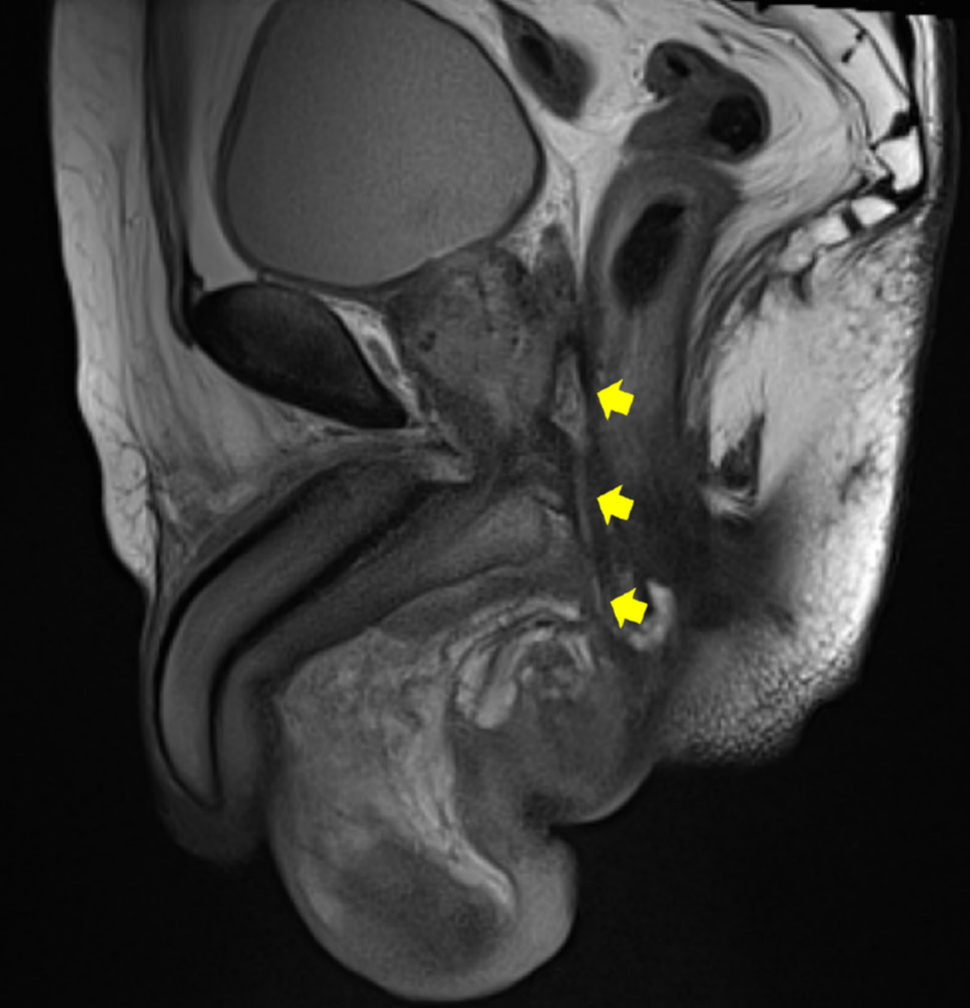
MRI showed tunnel‐like discharge of hydrogel from dorsal prostate to perineum.
